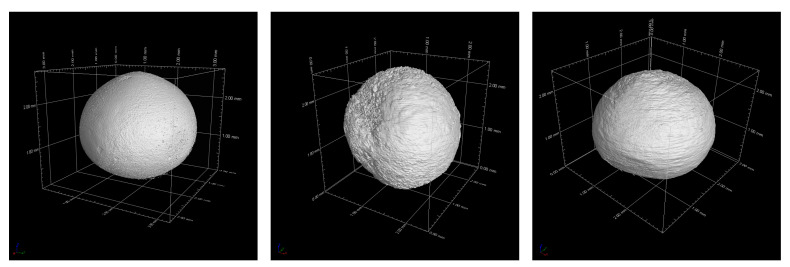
CT-rendered images of beads before compression test. Al_2_O_3__100-L (left), Nb_95-L (center), and Nb_100-L (right).
